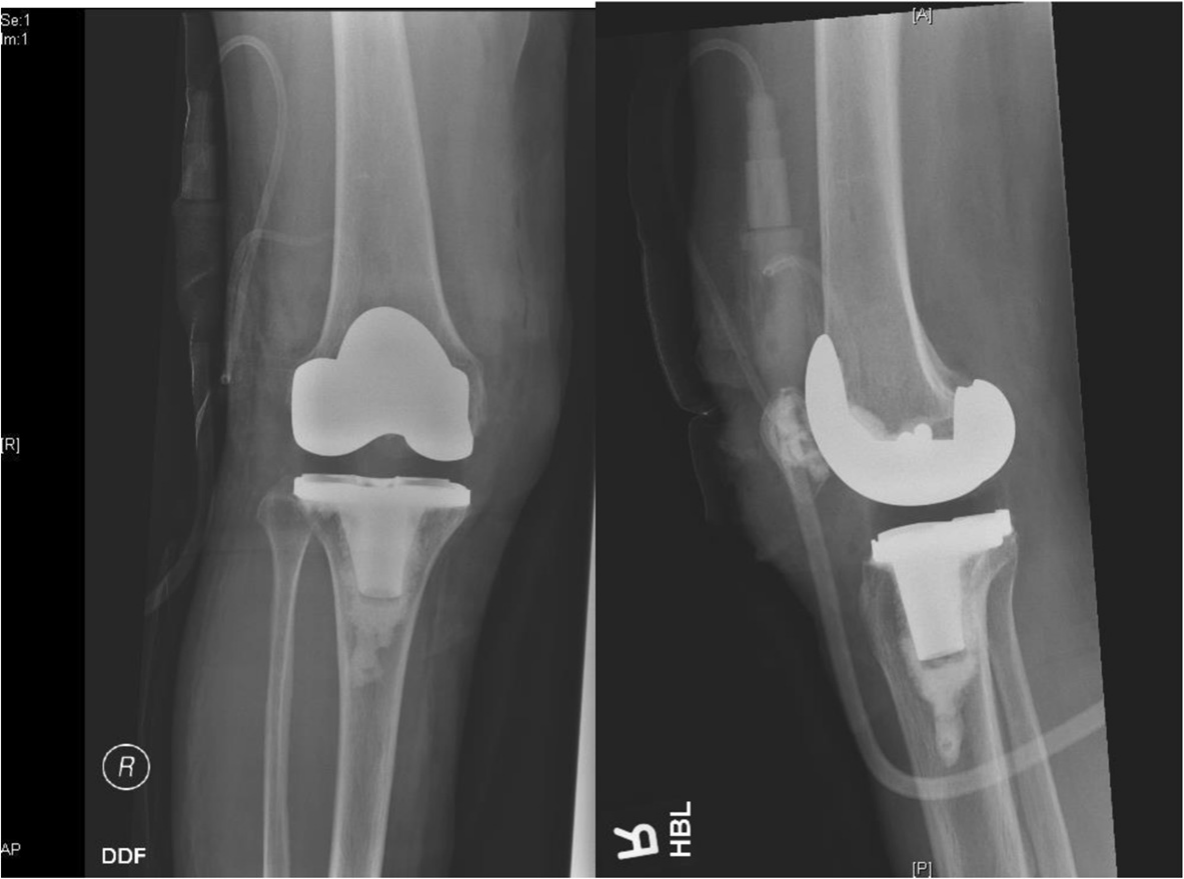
Post-operative check radiographs
